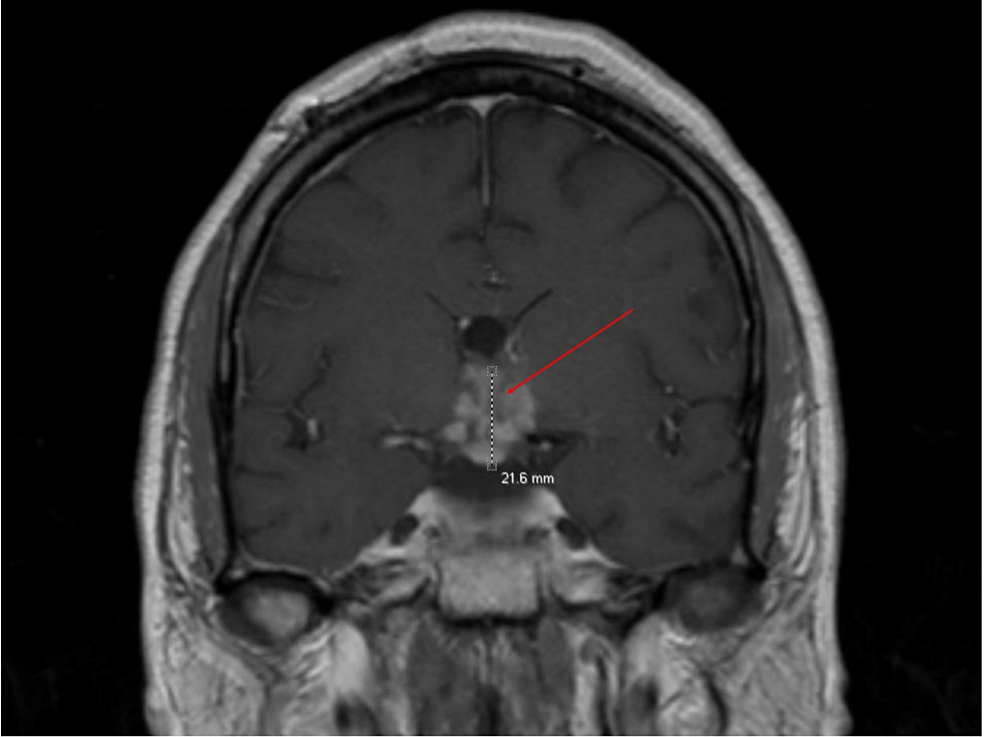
Coronal T1 post-contrast MRI pituitary showing a multilobulated compressing mass within the inferior third ventricle, hypothalamus, and optic chiasm
